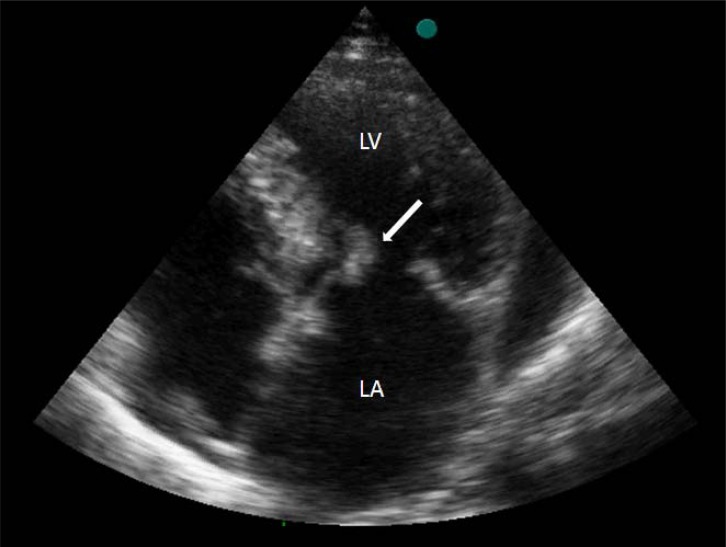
Transthoracic apical four-chamber view showing vegetation (arrow) on mitral anterior leaflet (LA: left atrium, LV: left ventricle).
